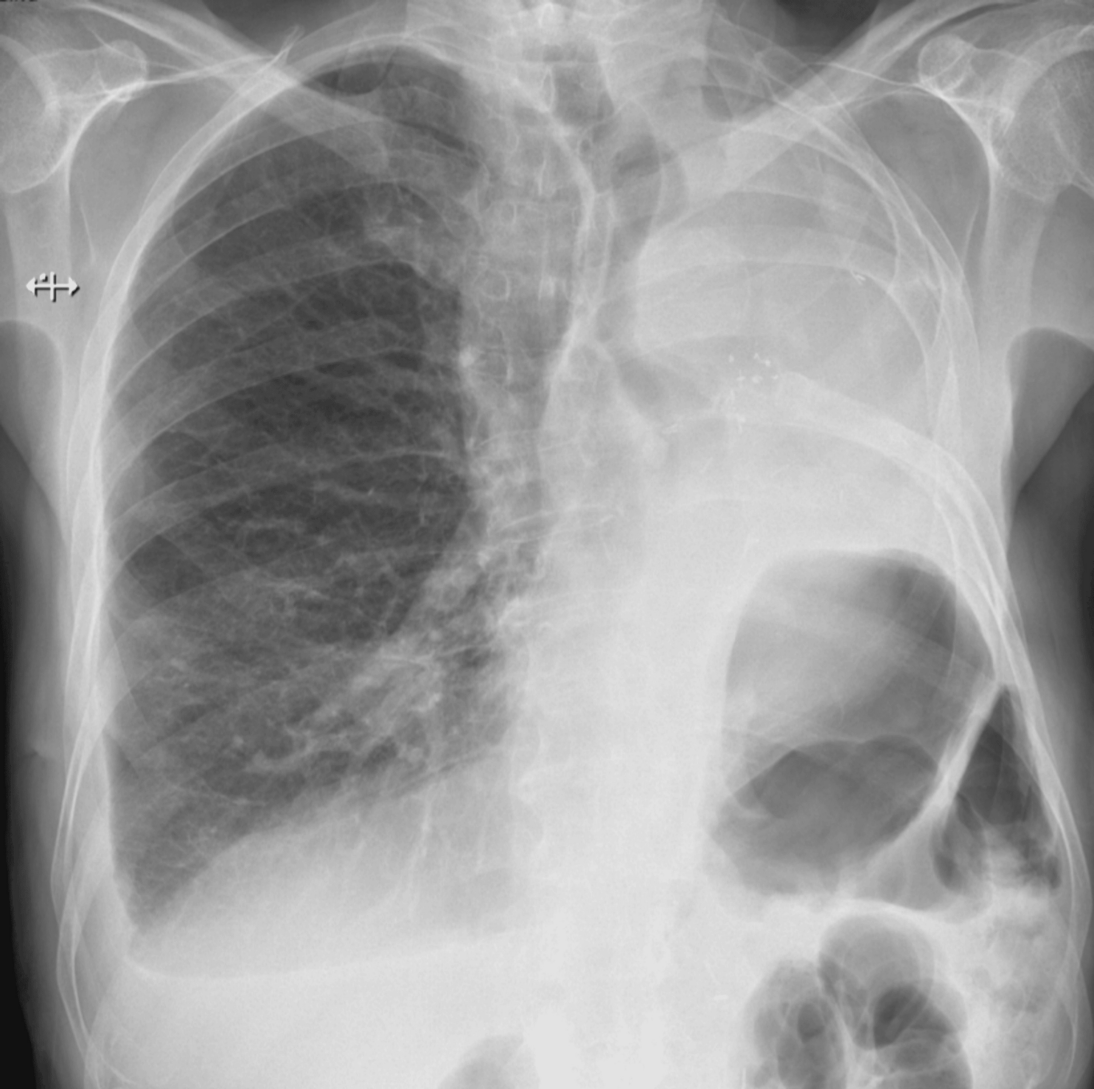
Chest radiograph one month after surgery. Chest radiograph obtained one month after surgery showing complete re-expansion of the right lung and a small residual postoperative pleural effusion, with no evidence of pneumothorax recurrence.
